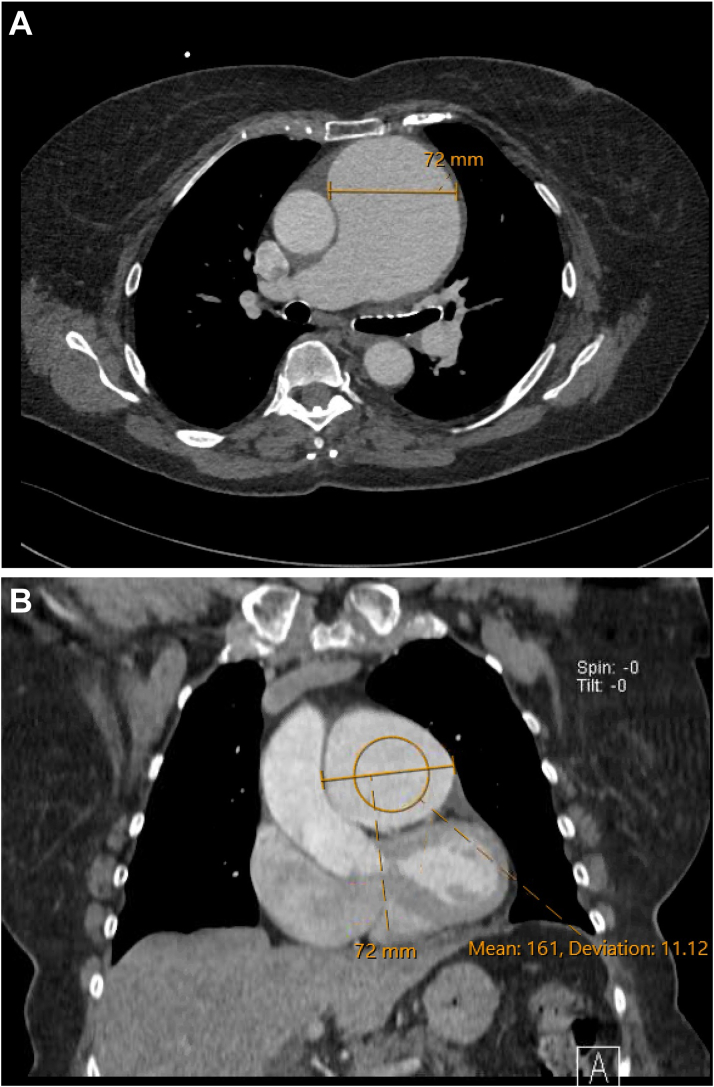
Findings on Contrast-Enhanced Chest Computed Tomography (A) Axial image shows the pulmonary artery aneurysm measuring 7.2 cm. (B) Coronal image shows the pulmonary artery aneurysm measuring 7.2 cm.
